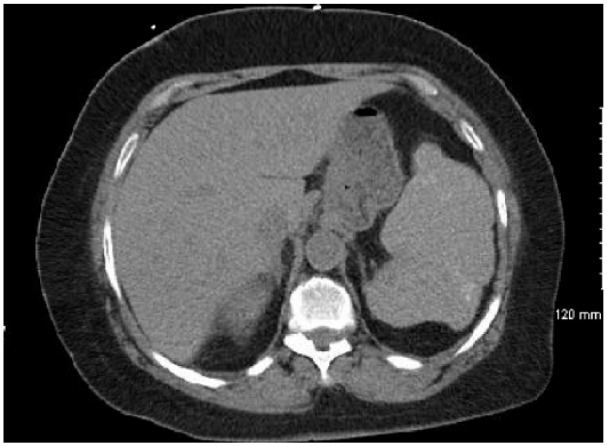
CT abdomen following red blood cell exchange transfusion demonstrated enlarged spleen consistent with acute splenic sequestration crisis.
